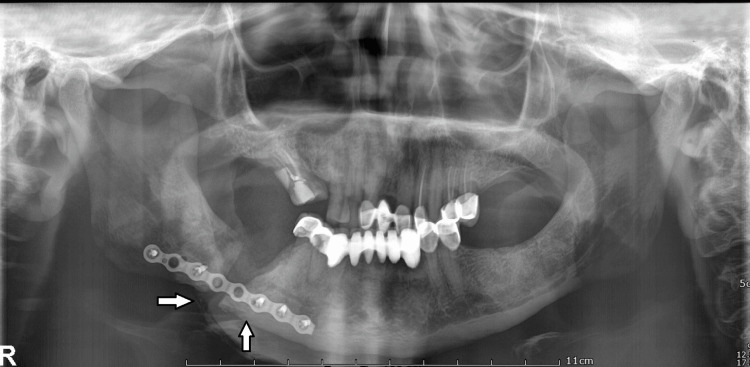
Panoramic radiograph showing fractured segments secured with an eight-hole titanium plate and screws.
